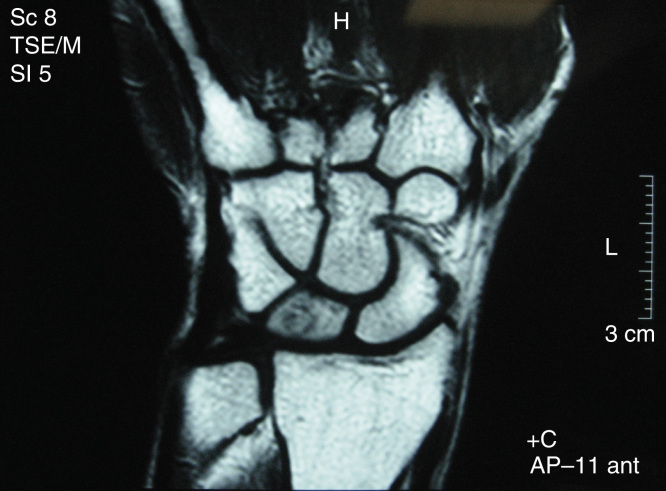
Preoperative computed tomography scan showing the patient’s wrist before second surgery. Nidus of the osteoma can easily be seen.
